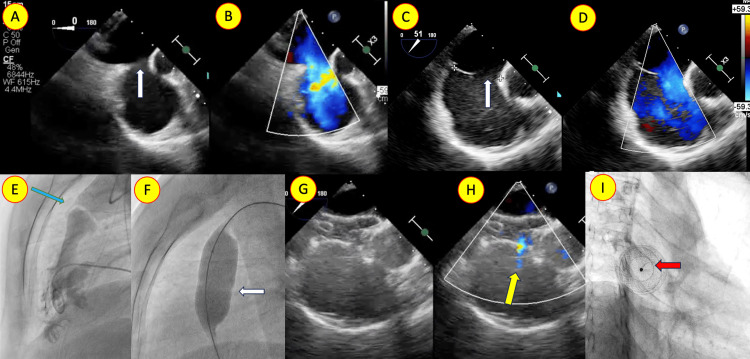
Transesophageal echocardiogram (TEE) in zero-degree view (A, B) and 50-degree view (C, D) with color Doppler showing the moderate secundum atrial septal defect (ASD) (white arrow) with absent aortic rim and thin floppy posterior rim. Angiogram in the lateral view showing (E) doming of the pulmonary valve (blue arrow) and disappearance of the waist during balloon dilatation (white arrow). (G) TEE after device closure with color Doppler (G, H) showing the stable ASD device and fenestration flowing left to right (yellow arrow). (H) Fluoroscopic image showing the fenestration (red arrow) in the oblique view.
